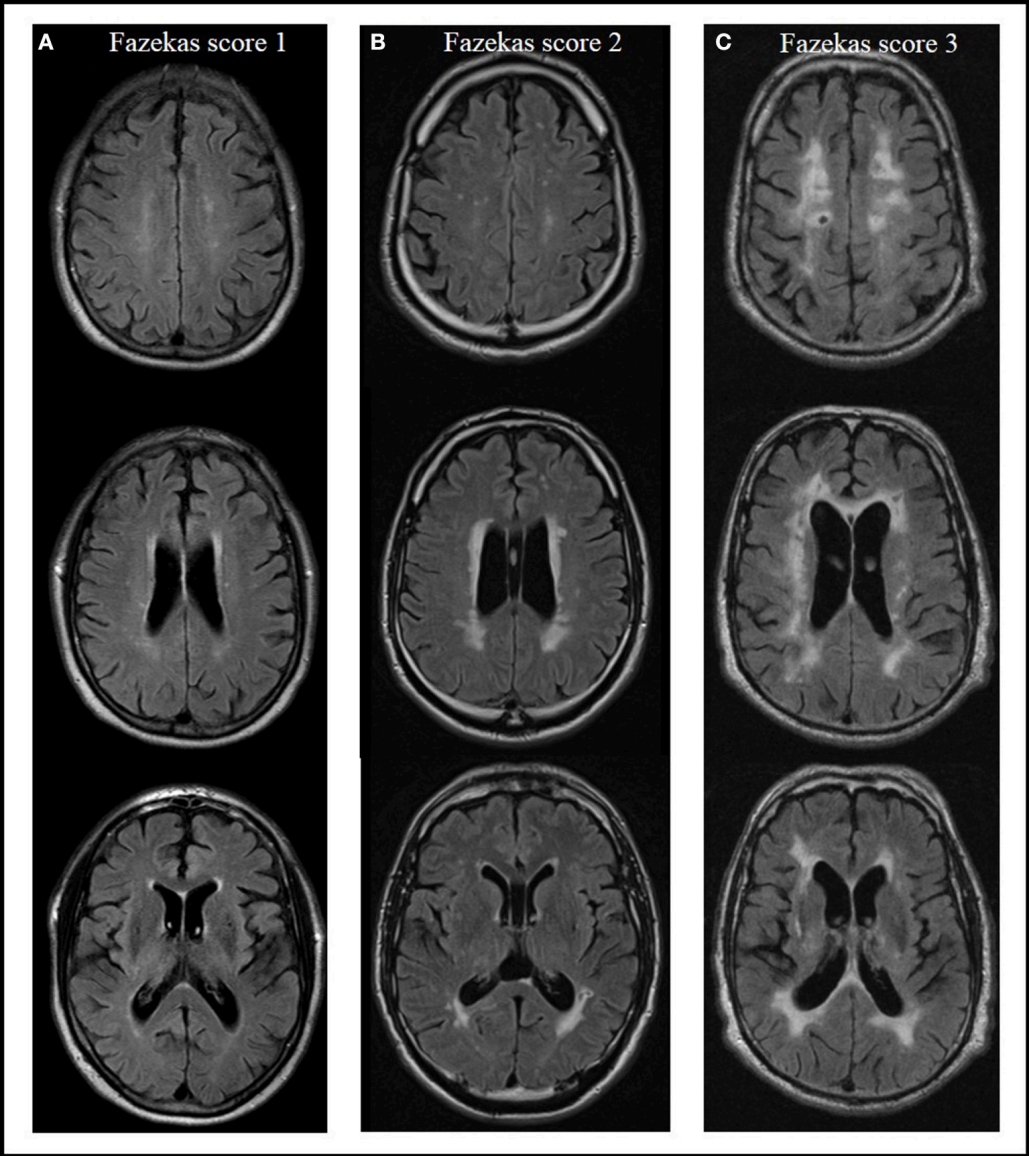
Presence and severity of white matter changes in MRI according to the Fazekas score, examples from the actual evaluation. Score 1 (mild) with focal or punctuate lesions (A); score 2 (moderate) with beginning confluent lesions (B); score 3 (severe) with confluent lesions (C) (Prins and Scheltens, 2015).
